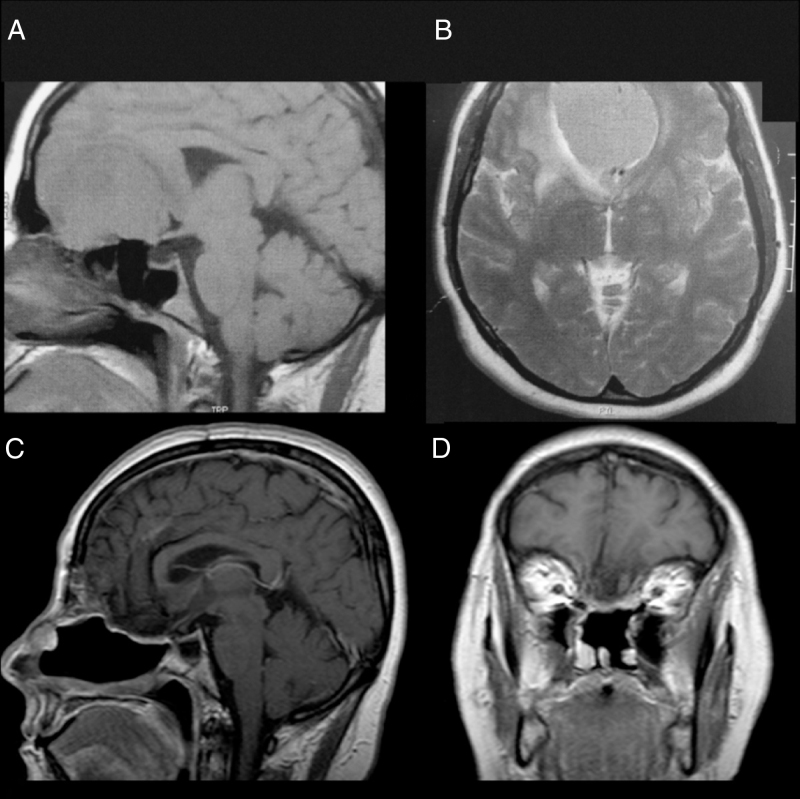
Patient 1, female, 49 years old. (A, B) Preoperative MRI without a contrast showing a large mass in the anterior fossa with mass effect. The anterior cerebral arteries are displaced posteriorly and there is invasion of the anterior and posterior ethmoid. (C, D) Postoperative 4 years MRI with contrast, showing gross total resection and no signs of tumour recurrence or brain herniation. Both flaps can be seen with gadolinium enhancement and preservation of the pedicles and viability of the endoscopic double flap.
